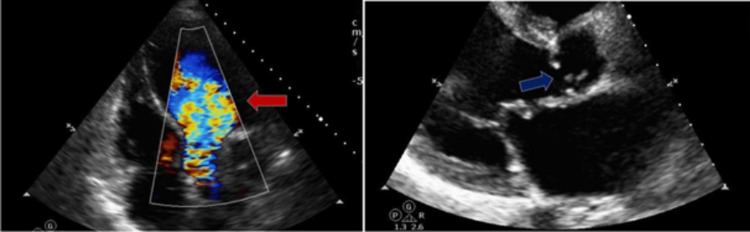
TTE with apical 5 chamber view (left) indicating the AR jet (red arrow); parasternal long axis view indicating the malcoaptation of the aortic valve leaflets (blue arrow)
